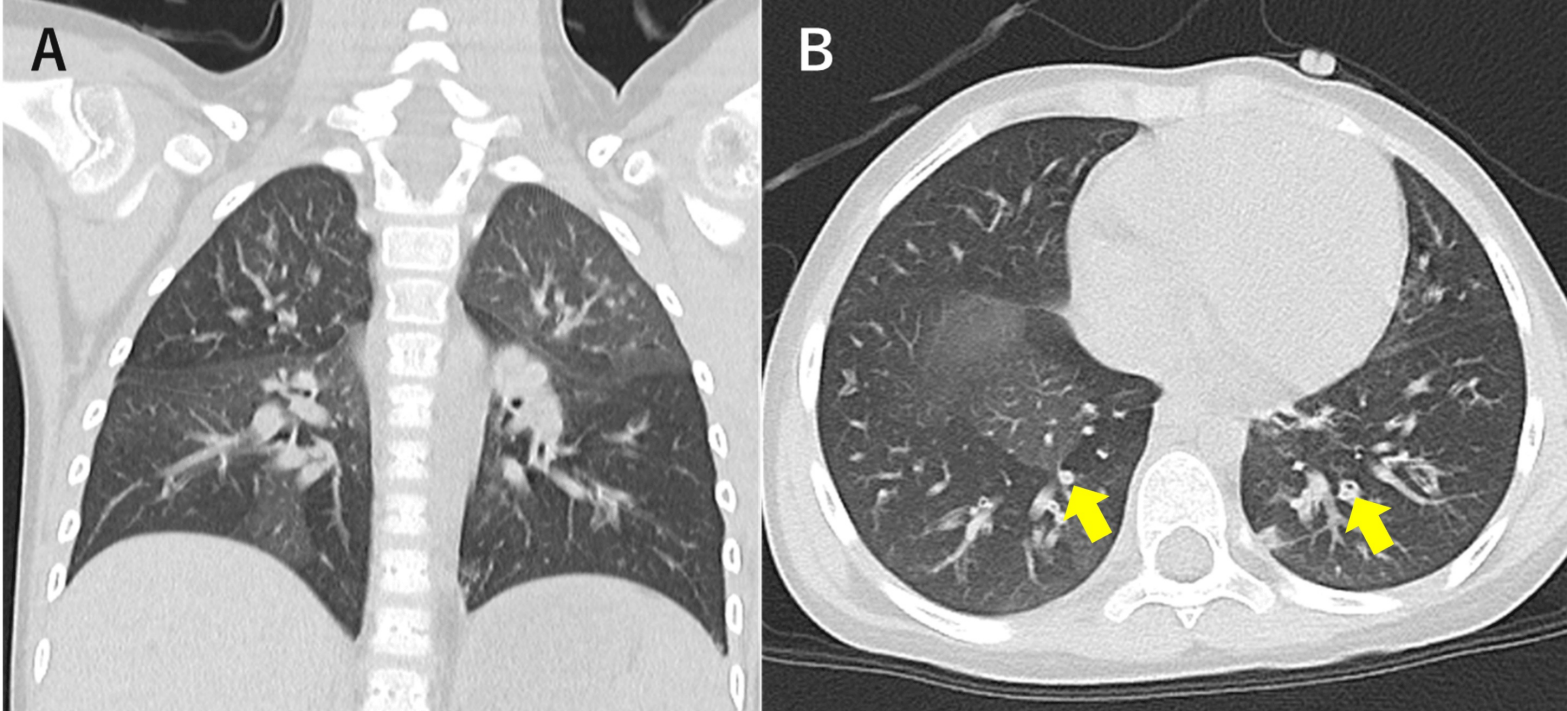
Chest CT demonstrating mosaic attenuation with air trapping Coronal (A) and axial (B) chest CT obtained on day 25 after autologous HSCT show a mosaic attenuation pattern with air trapping, corresponding to areas of bronchial wall thickening (arrows). No ground-glass opacities or centrilobular nodules are observed CT: computed tomography; HSCT: hematopoietic stem cell transplantation
